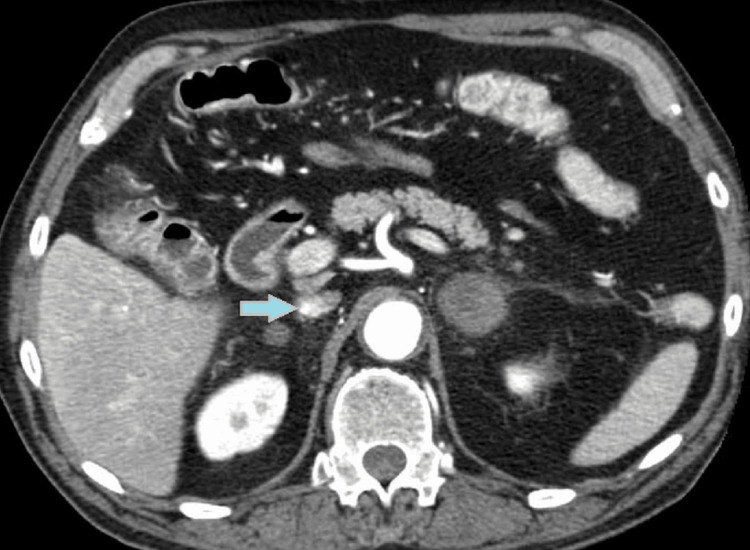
CT scan of the abdomen showing right adrenal hemorrhage (Blue arrow with yellow margin).
